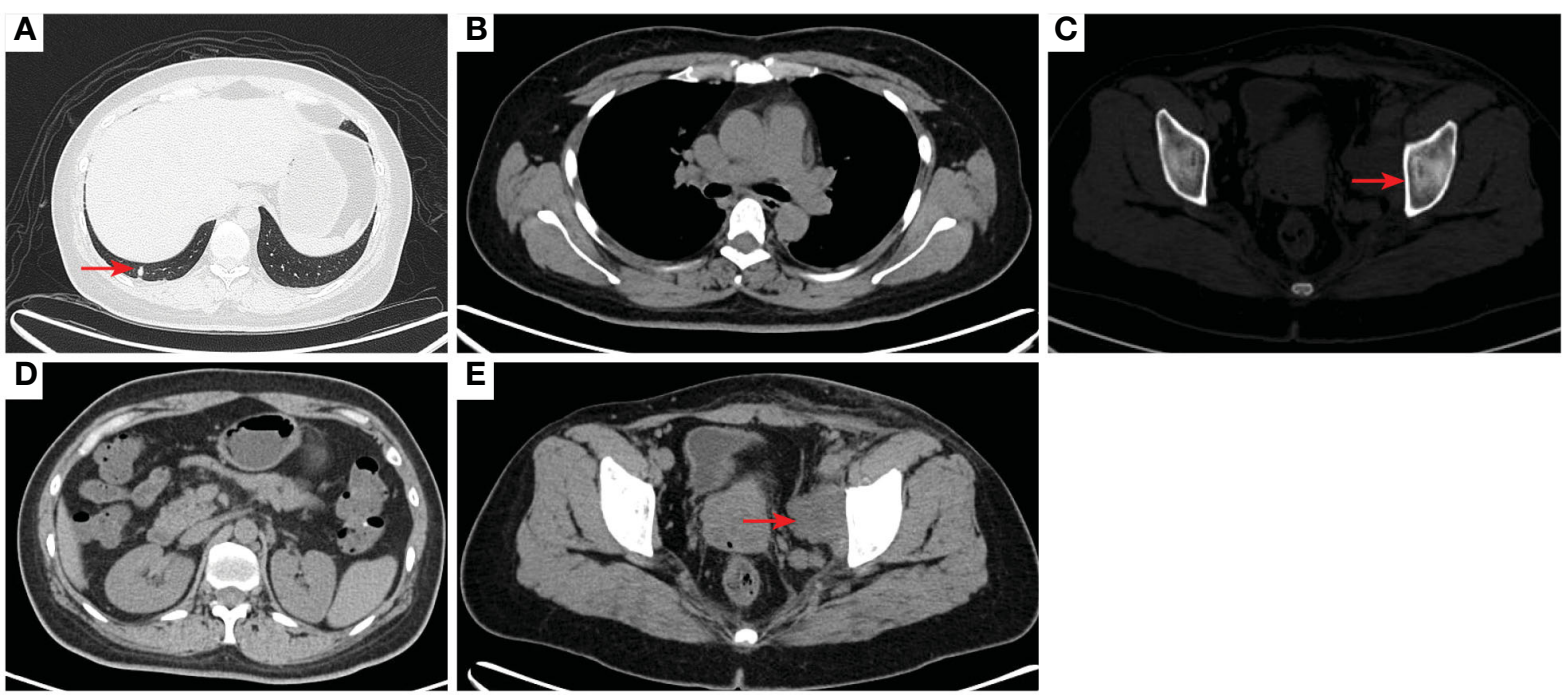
2020–7-10 CT scan: the metastatic tumor in the right lower lobe of the lung and the long diameter was 0.69 cm [red arrow, (A)]. Mediastinal lymph nodes were not significantly enlarged (B). Left acetabular metastases [red arrow, (C)]. Abdominal and abdominal lymph nodes were not significantly enlarged (D) No enlarged lymph nodes were found in the right hilum and the retroperitoneal area. The left pelvic lymph nodes were found and the short diameter was 4.05cm [red arrow, (E)].
